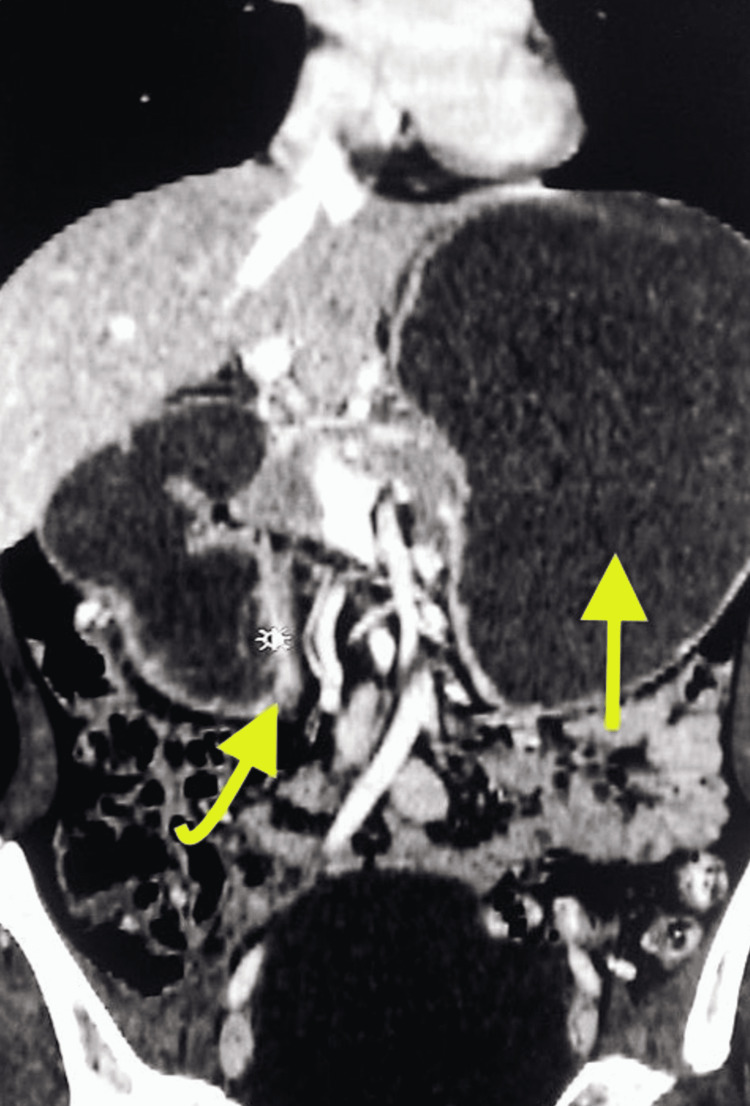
Computed tomography scan of the abdomen in coronal image showing dilated stomach (straight arrow) and dilated second portion of the duodenum (curved arrow with pointer).
